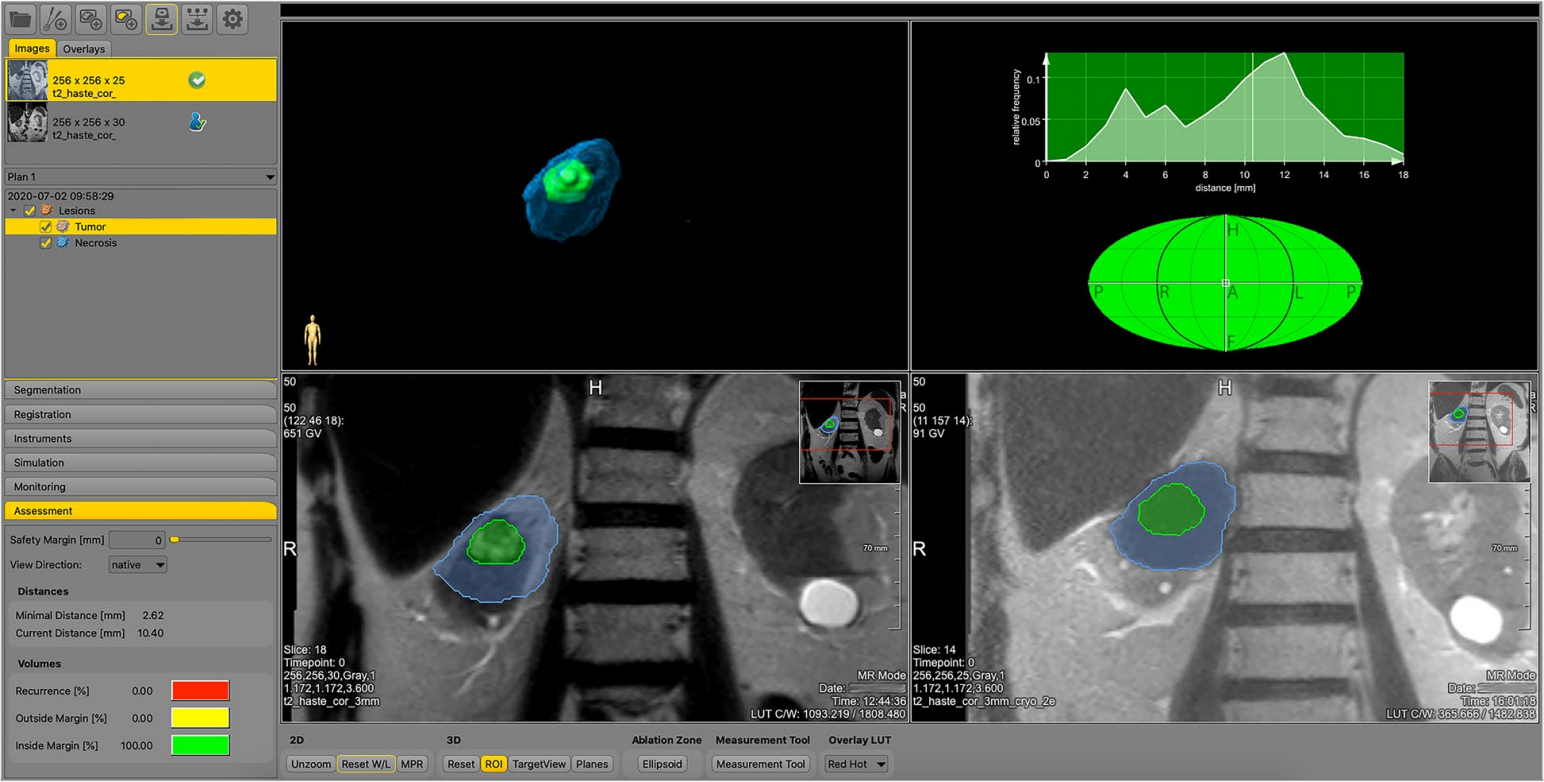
Overview of Software Assistant for Interventional Radiology (SAFIR) layout. Available image series and tools are shown in bars on the left. Image views from left to right, top to bottom: (1) 3D view of annotated ice ball (blue) and tumor (green) overlap, (2) tumor divided in octants along the left-right, cranio-caudal and anterior-posterior axes showing color-coded subareas of total tumor volume with complete coverage including user-defined treatment margin (green), complete coverage but insufficient margin (yellow) and incomplete coverage (red)., (3) pre-ablation and (4) post-ablation T2-haste coronal MRI-images showing the tumor (green) and co-registered ice ball (blue) volumes. Side bar on the left shows calculated 3D ablation margin metrics
